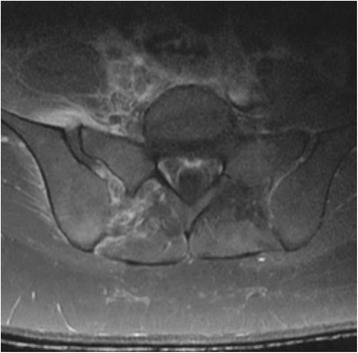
Magnetic resonance imaging of the pelvis showing widening of the patient's right sacroiliac joint space with ‘increased’ fluid in the joint. Also visible are associated inflammatory changes in the surrounding musculature, indicative of an inflammatory process occurring in the joint
